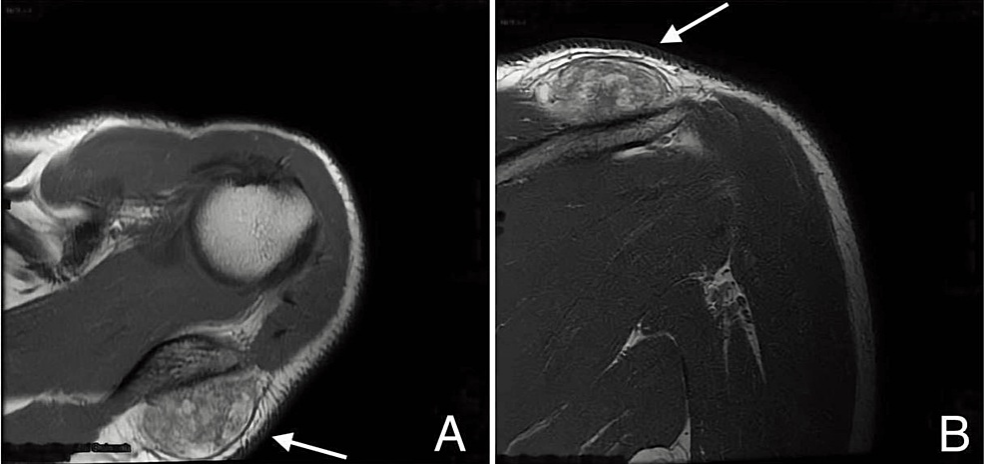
T1 MRI of the shoulder (A) Axial view.(B) Coronal view.
